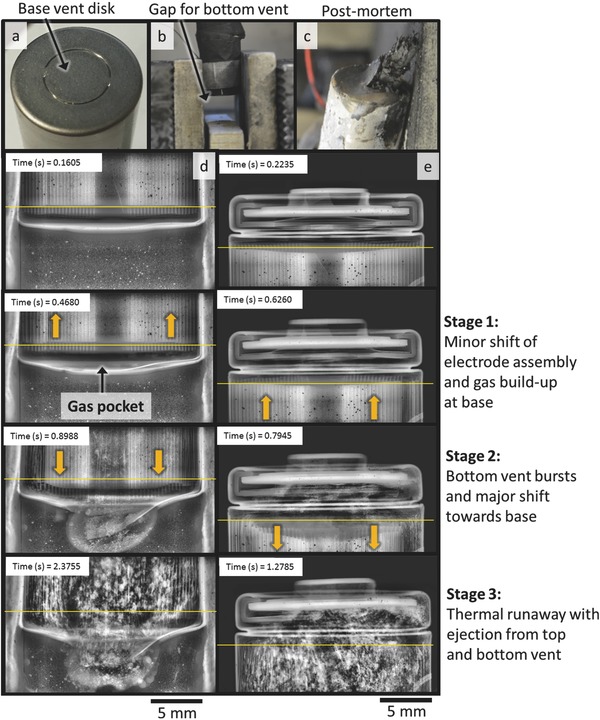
a) Photograph showing the vent disk at the base of the 18650 prototype cell. b) Photograph showing the position of the 18650 cell with clearance at its base for the second vent. c) Postmortem photograph showing ruptured vent disk. d) Time‐stamped radiographs showing the activation of the secondary vent during thermal runaway. e) Time‐stamped radiographs showing the crimp‐region of the 18650 cell during thermal runaway. Radiography movies of (d) and (e) are provided as Movies S14 and S16, respectively, in the Supporting Information.
